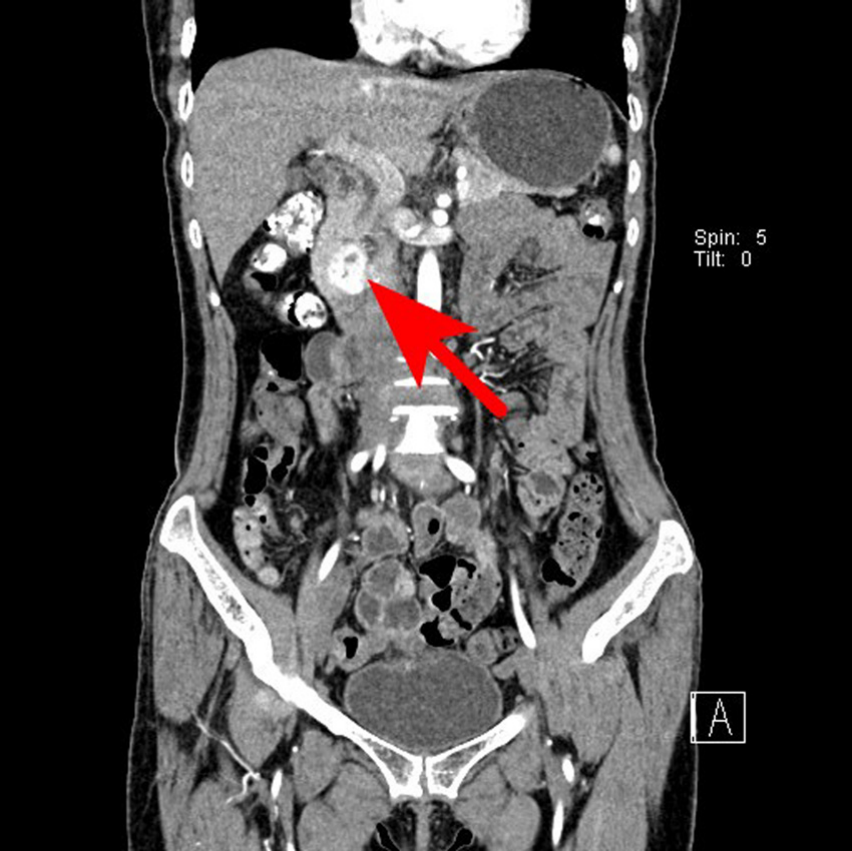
Coronal scan of the arterial phase. The lesions showed uneven enhancement, the highest CT value reached 192 HU, and the low-density areas were not enhanced.
